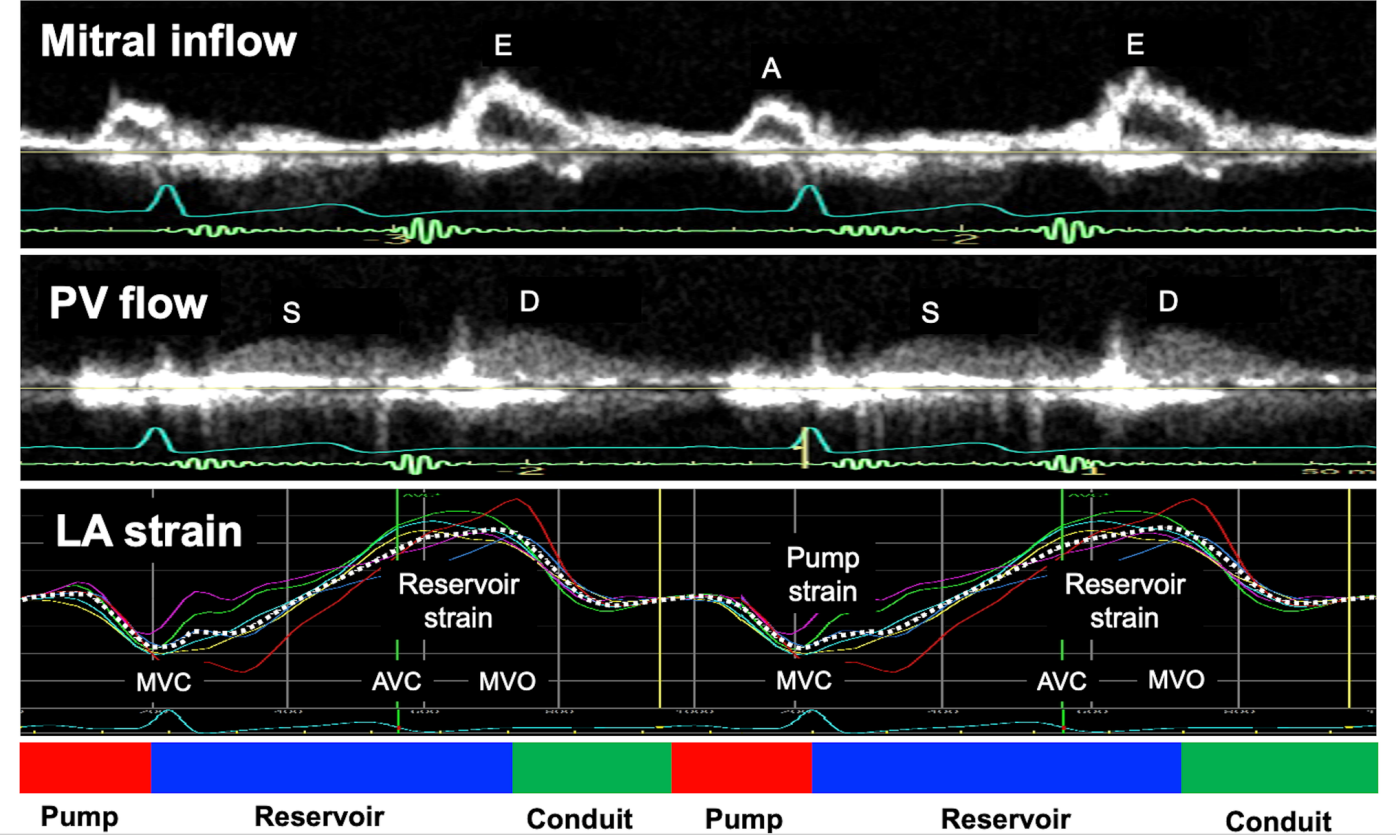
Doppler signals of mitral inflow and pulmonary venous velocities and LA strain curve. The figure demonstrates two Doppler signals of mitral inflow and pulmonary venous flow, as well as LA strain curve simultaneously. LA, left atrial; PV, pulmonary venous; MVC, mitral valve closure; AVC, aortic valve closure; MVO, mitral valve opening
